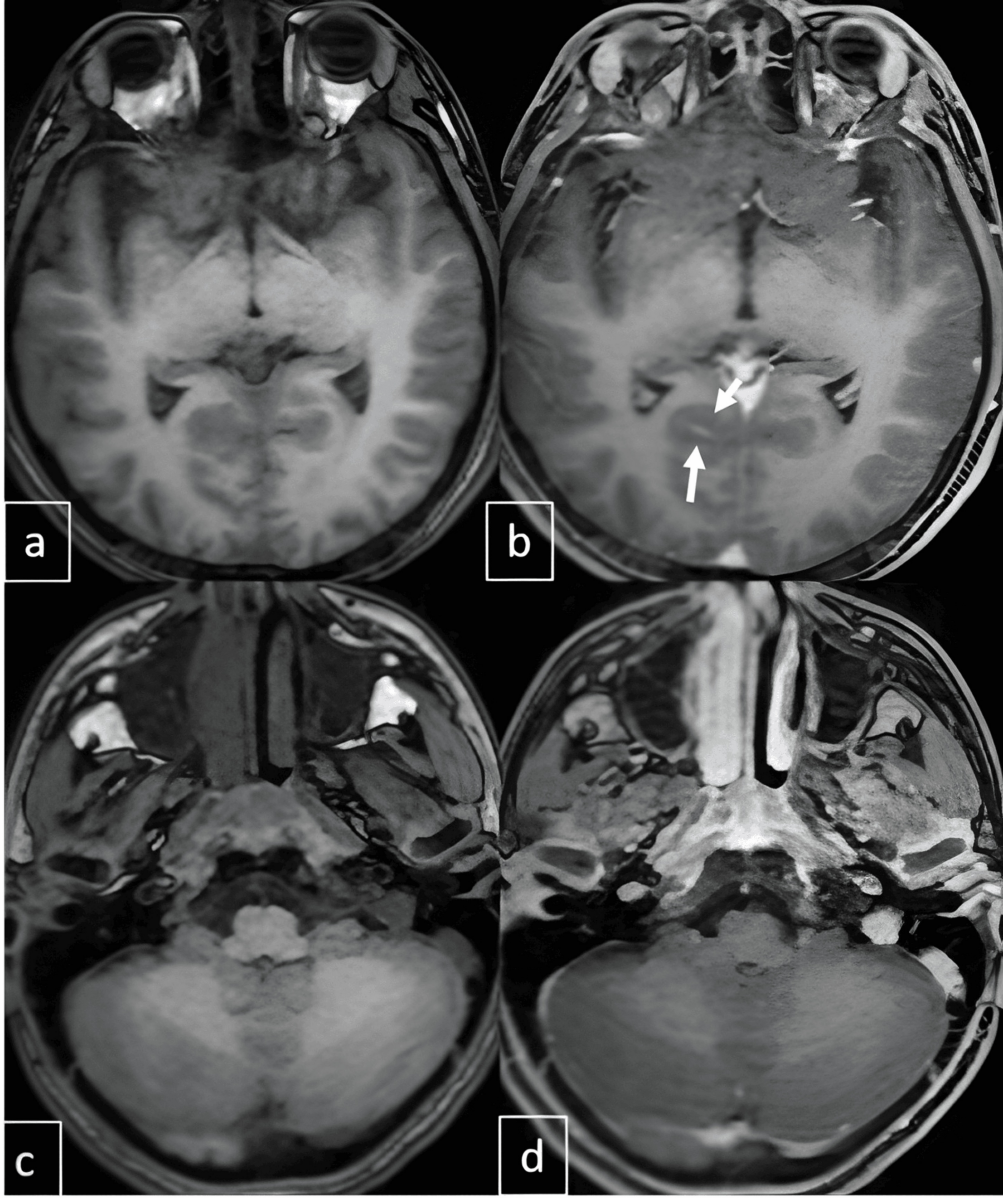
On axial pre-contrast (a and c) and post-contrast (b and d) T1-weighted images, no significant enhancement is observed within the cerebellar parenchyma; however, leptomeningeal enhancement is evident in the right posterior parahippocampal region, as indicated by the arrow (b).
